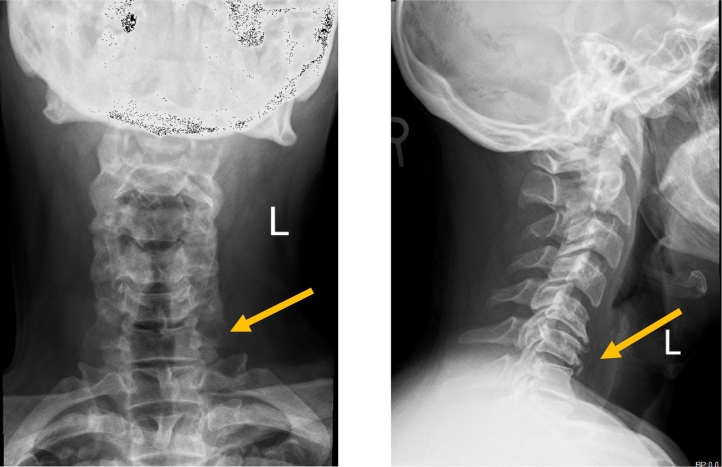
X-ray antero-posterior and lateral views of cervical spine showing C5–C6 and C6–C7 anterior and posterior vertebral osteophyte formation with loss of disc height, as well as kyphotic deformity of cervical spine.
